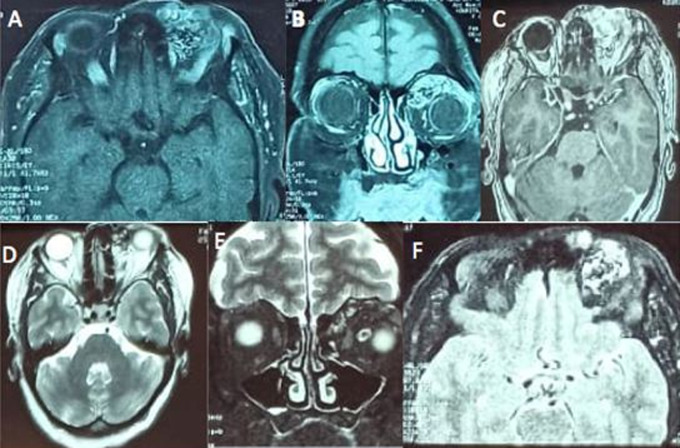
(A) orbito encephalic MRI; (B) axial and coronal T1 section: image of orbital mass in upper internal angle of left orbit, the lesion has an in heterogeneous hypersignal with serpiginous appearance; (C) axial T1 section with gadolinium enhancement, the mass has intense contrast enhancement; (D, E, F) axial and coronal T2 section, axial diffusion section: heterogeneous hypersignal lesion with serpiginous appearance mimicking an arteriovenous malformation
